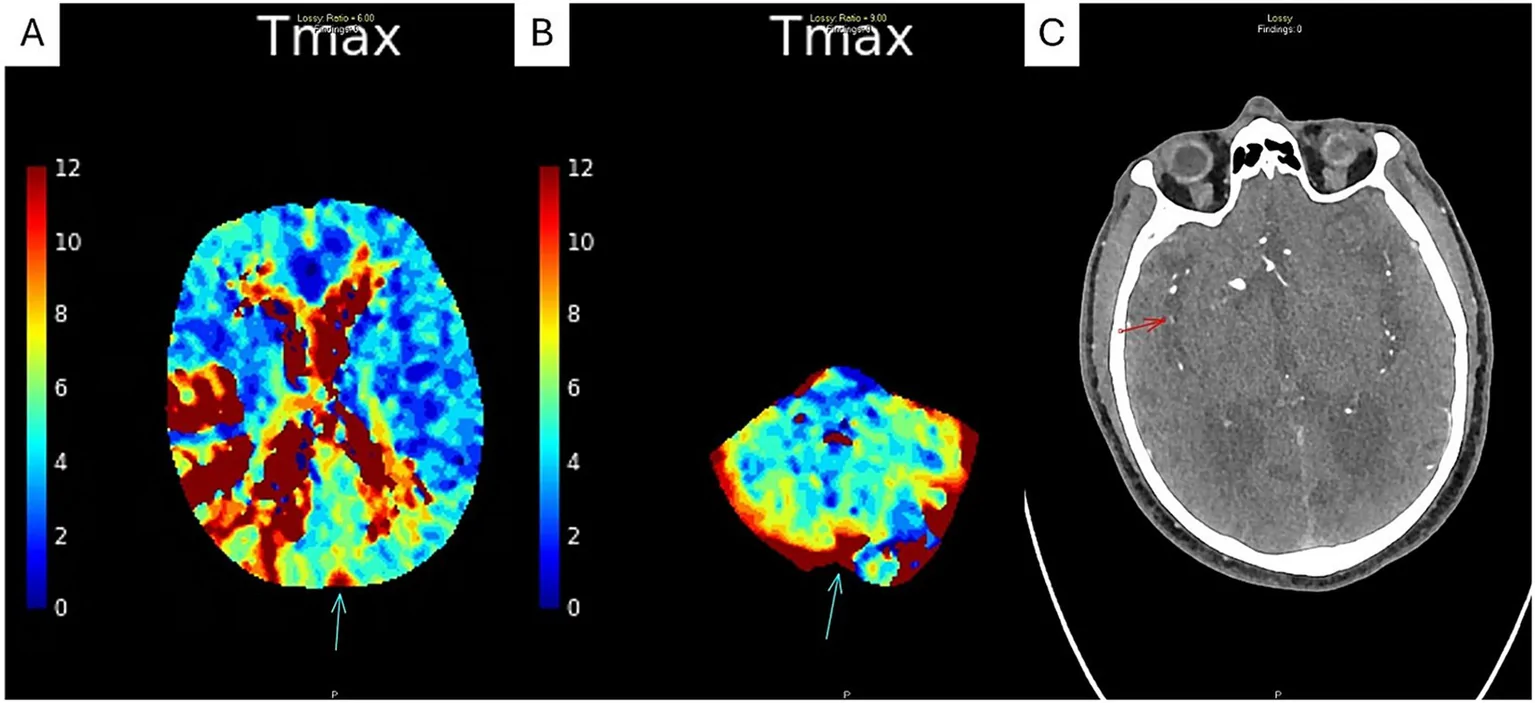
Pre-treatment CTP demonstrating PVT+. Imaging from an elderly male presenting with acute right M2 occlusion. His admission National Institutes of Health Stroke Scale score was 19. The 90-day modified Rankin Scale score was 6. Tmax perfusion maps showing prolonged venous transit in (A) the superior sagittal sinus (blue arrow) and (B) the torcula (blue arrow). Computed tomography angiography (C) highlighting the right M2 occlusion (red arrow).
